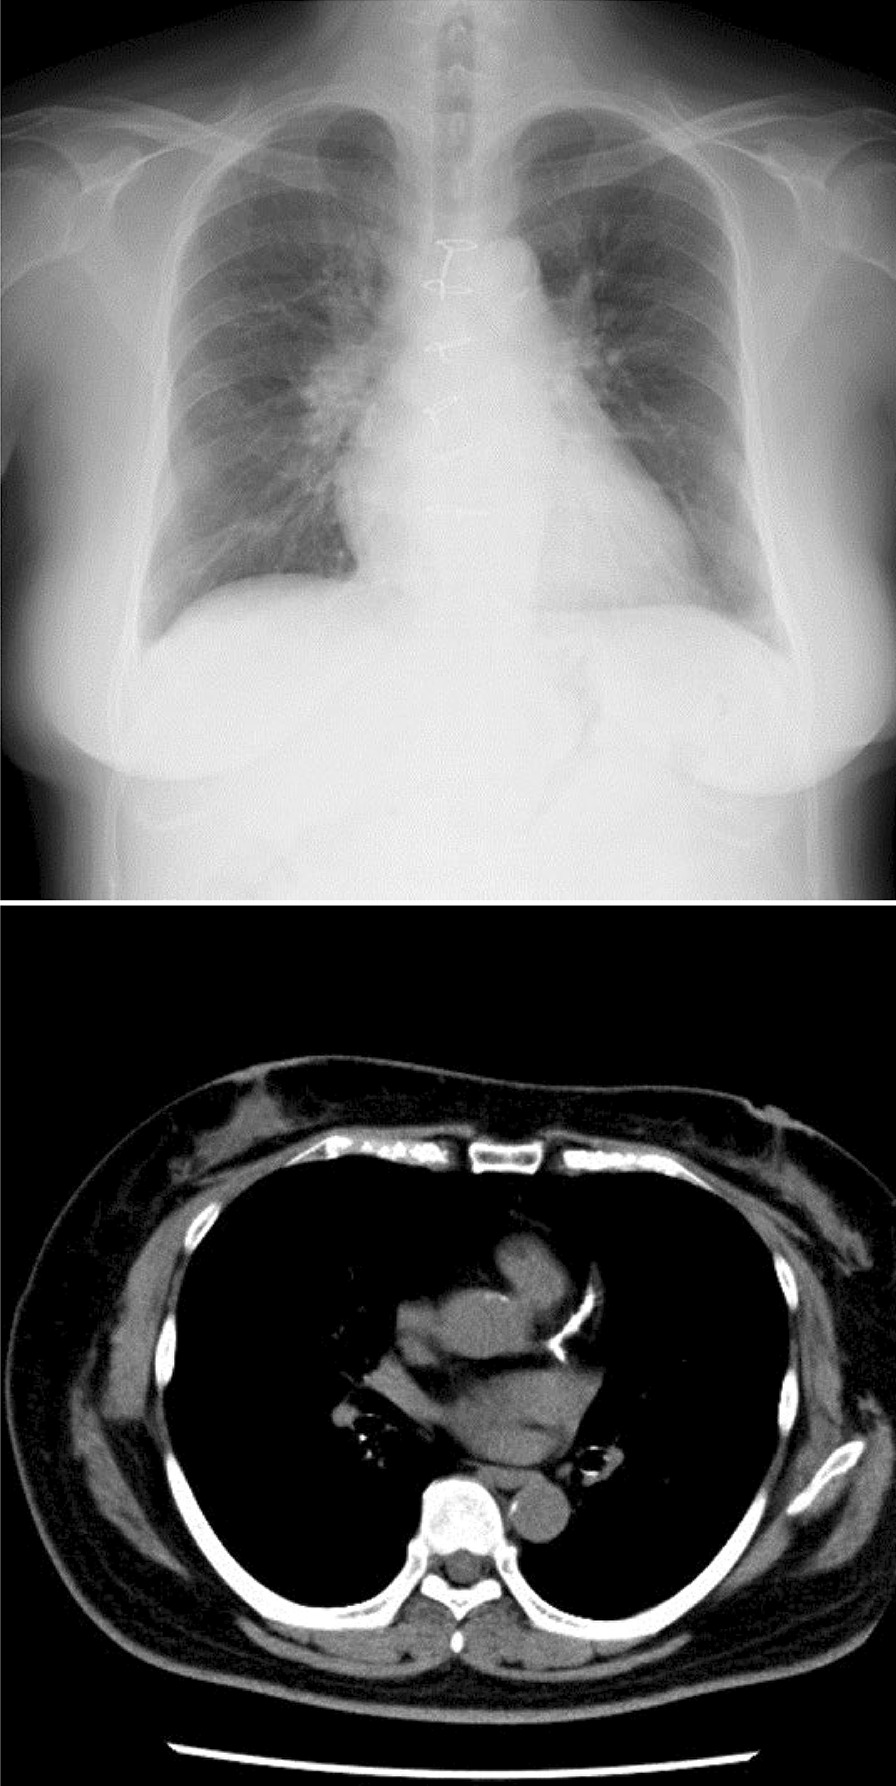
Preoperative chest radiography and computed tomography in Case 1. Chest radiograph showing a cardiothoracic ratio (CTR) of 57%, and computed tomography (CT) demonstrates a severely calcified coronary artery
